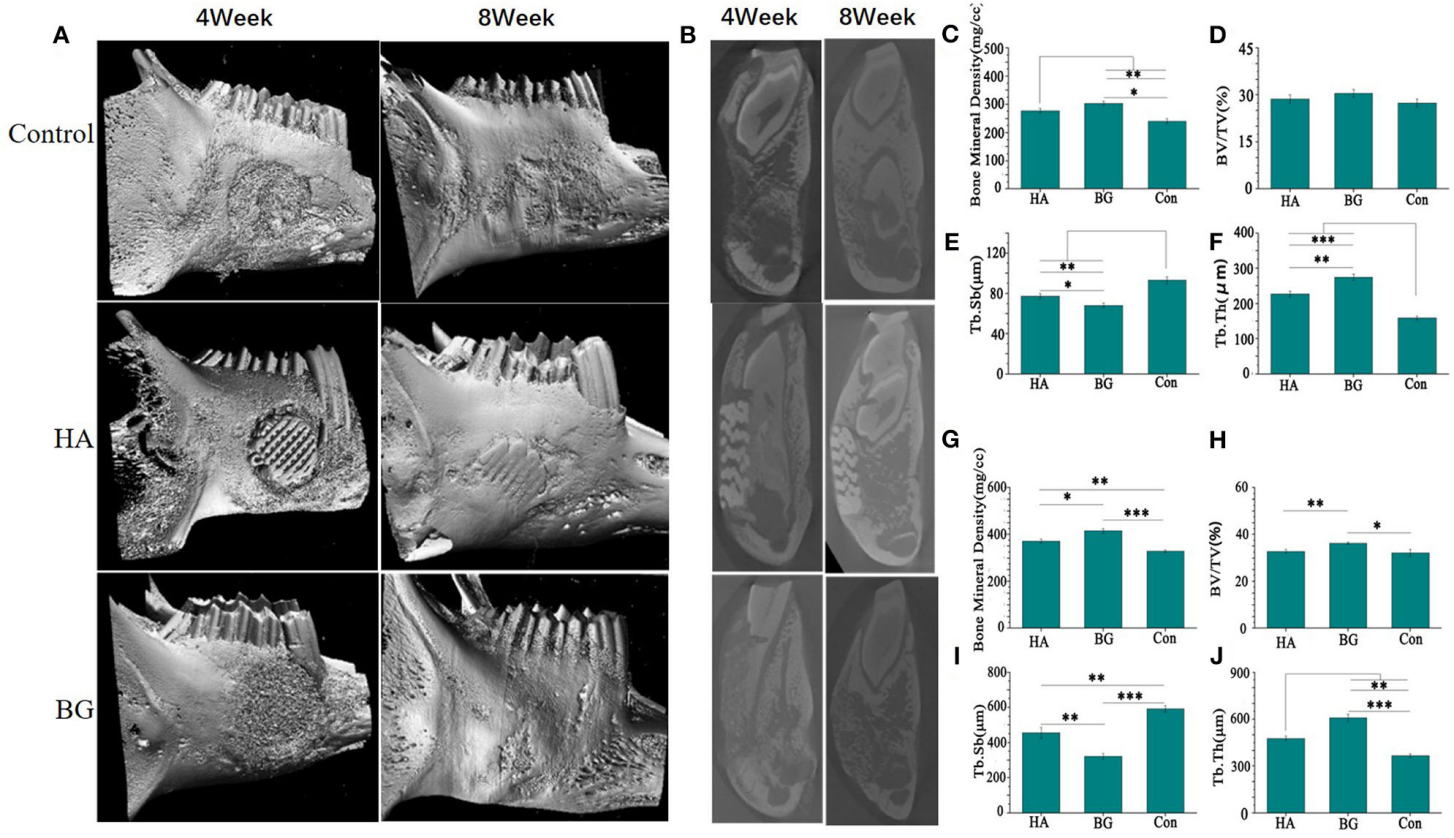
The CT scan image(A) and sagittal image (B) of the harvested mandible healed for 4 weeks and 8 weeks; The bone mineral density (BMD) after 4 weeks (C) and 8 weeks (G); The bone volume density (BV/TV) after 4 weeks (D) and 8 weeks (H); The trabecular separation, (Tb.Sp) after 4 weeks (E) and 8 weeks (I); The trabecular thickness(Tb.Th) after 4 weeks (F) and 8 weeks (J). P* < 0.05, *P* < 0.01, and P* < 0.001.
